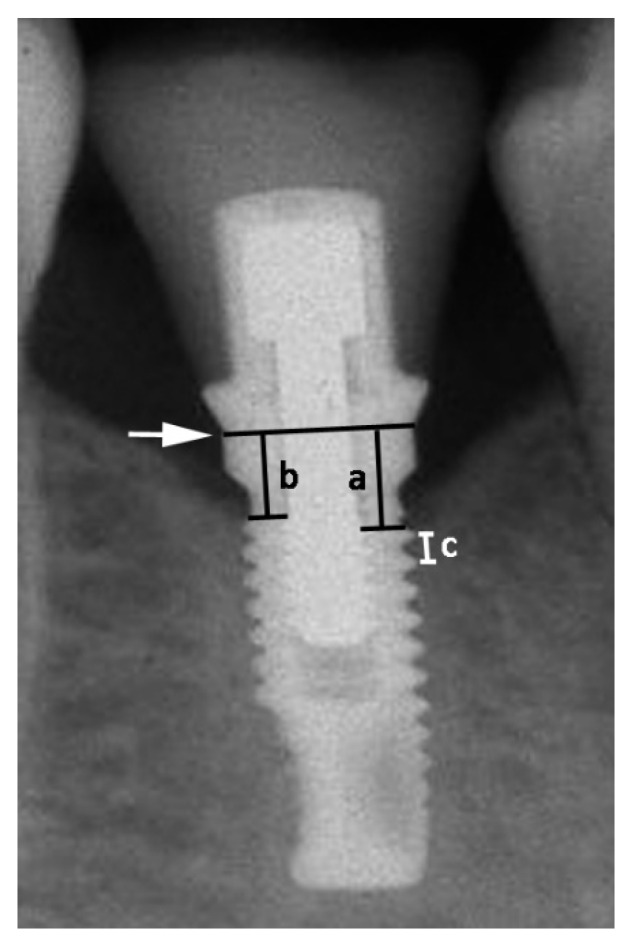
Measurement of the distance from the implant-abutment junction (black line indicated by the white arrow) to the first visible bone-to-implant contact, on both mesial (a) and distal (b) sides, on periapical radiographs. Calibration was based on the inter-thread distance of the implants (c).
